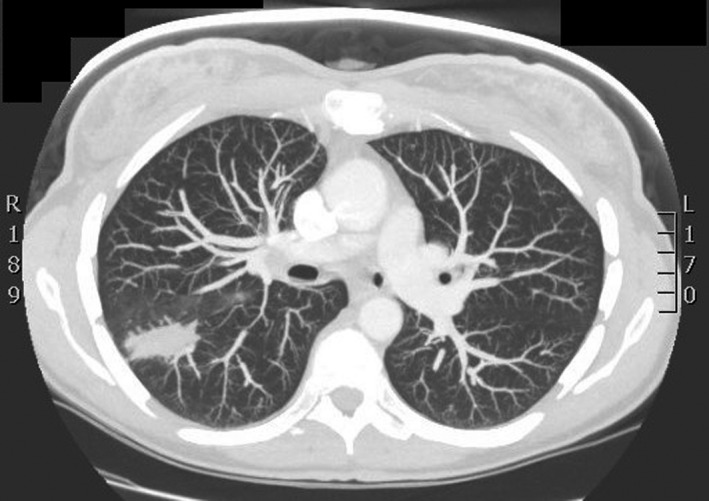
Inflammatory pseudotumor contrasted CT chest
